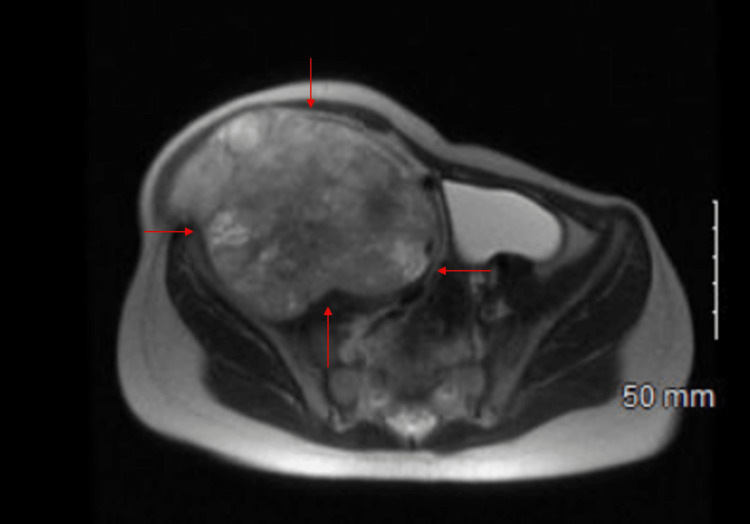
Axial T2-weighted MRI without contrast Rhabdomyosarcoma (RMS) is delineated by the arrows.
